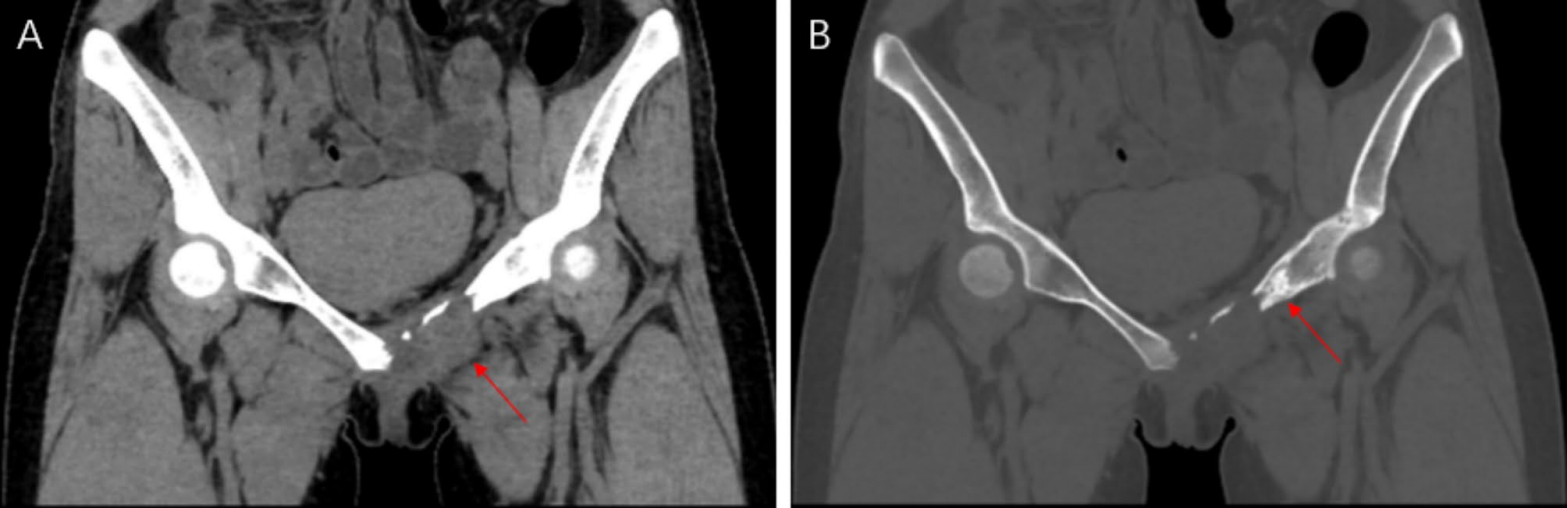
CT revealed a lesion of osteolytic bone destruction in the left pubis with a soft tissue mass (A, arrow), surrounded by local reactive osteosclerosis (B, arrow). And the bone cortex surrounding the lesion was damaged
